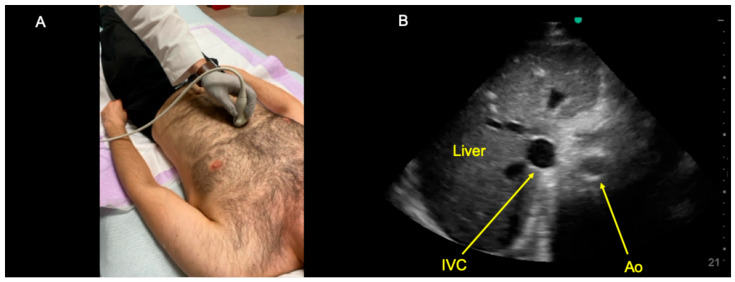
(A) Probe positioning used to obtain the subxiphoid short-axis view of both the inferior vena cava (IVC) and abdominal aorta (Ao). A low-frequency transducer is placed in the subxyphoid region in the transverse plane at the patient’s midline, with the probe indicator pointing toward the patient’s left side. The ultrasound probe is placed approximately perpendicular to the patient’s skin, and the beam is fanned cranially or caudally until the IVC and aorta are visualized in the short axis as shown in the right panel. (B) Example of a short-axis view of the abdominal aorta and IVC. As shown in this image, this short-axis view of both vessels provides useful data above and beyond what the long-axis view is capable of showing. Specifically, the short-axis view of these two great vessels (1) allows one to appreciate the visual differences between the two vessels in each individual patient; (2) permits recognition of lateral motion of the IVC relative to the transducer (a phenomenon that is difficult to distinguish from true IVC collapsibility when viewed solely from the IVC long-axis view); and (3) immediately reveals the location of the IVC in any given patient, facilitating the acquisition of the IVC long-axis view.
